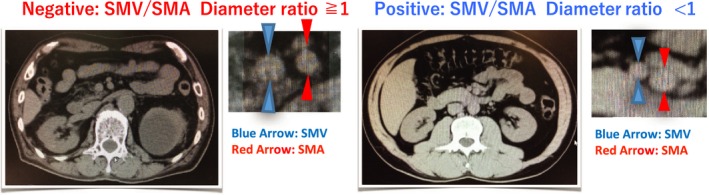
Plain computed tomography (CT) images of the smaller superior mesenteric vein (SMV) sign for the detection of acute superior mesenteric artery (SMA) occlusion. The SMA and SMV exist side‐by‐side in the upper part of the kidney. Left panels, CT images showing a negative smaller SMV sign, suggesting normal perfusion of the intestine. Right panels, CT images showing a positive smaller SMV sign, suggesting hypoperfusion of the mesenteric artery.
